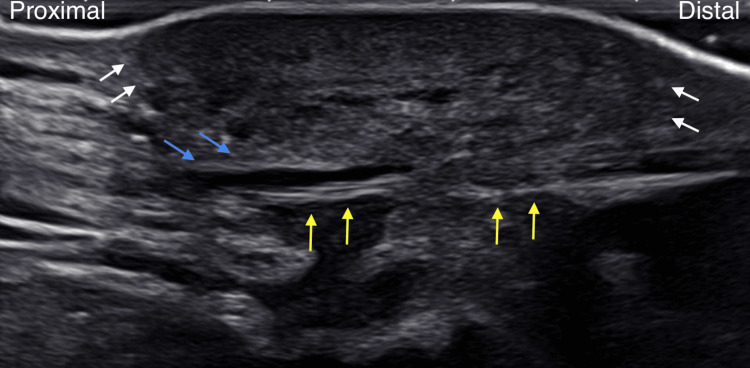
Long-axis ultrasound of the left anterolateral ankle showing a heterogenous hypoechoic mass with irregular margins (white arrows) anterior to the extensor retinaculum (blue arrows pointing to the superior band of the extensor retinaculum and yellow arrows pointing to inferior band).
